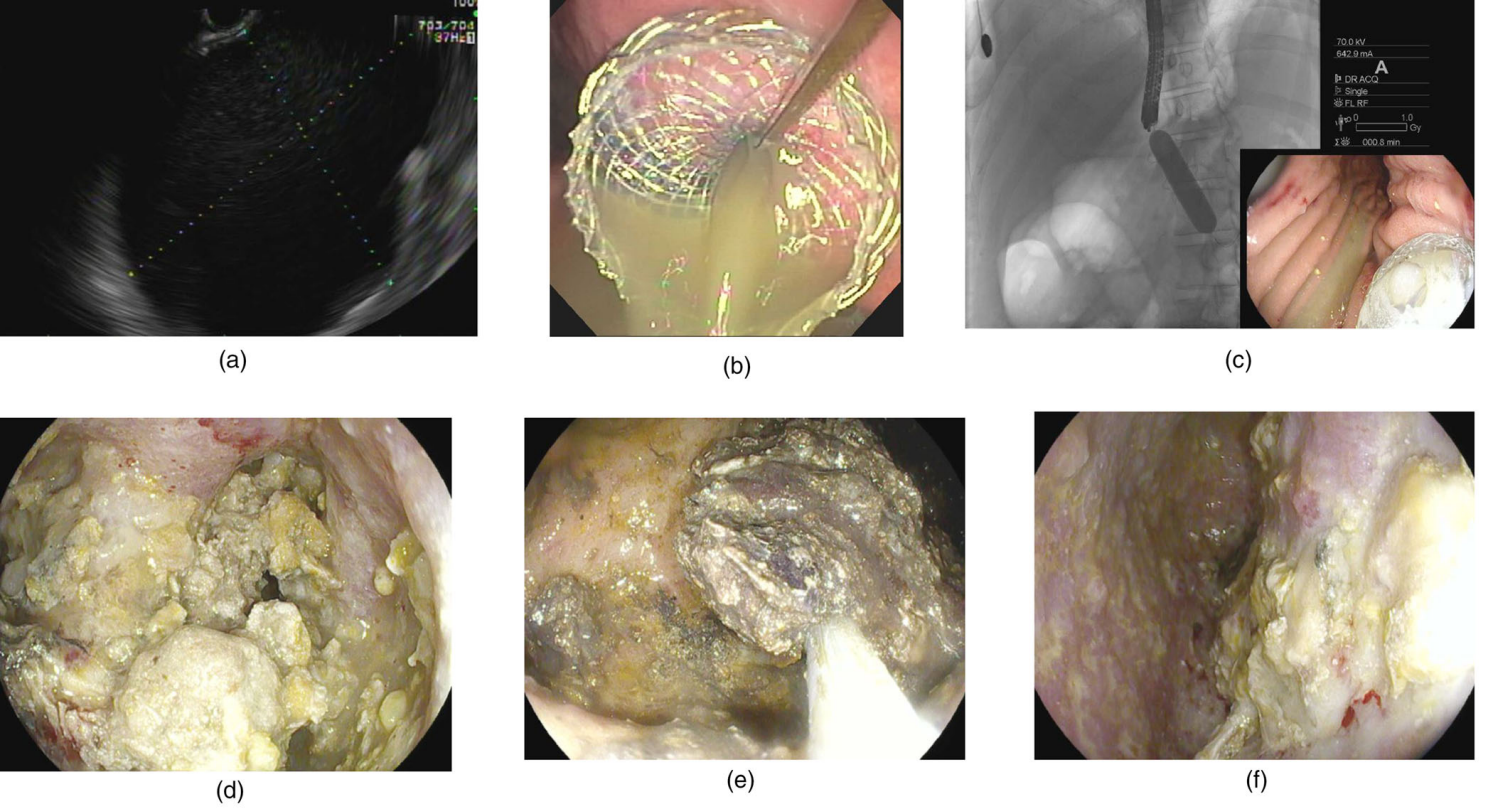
Endoscopic management of walled‐off necrosis. (a) Endoscopic ultrasound imaging of walled‐off necrosis. (b) Endoscopic visualization of lumen apposing metal stent after deployment in the cyst cavity with drainage of purulent fluid. (c) Balloon dilatation of lumen apposing metal stent after deployment under endoscopic and fluoroscopic guidance. (d) Endoscopic visualization of necrosis in the cyst cavity prior to direct endoscopic necrosectomy. (e) Direct endoscopic necrosectomy using a snare. (f) Clean cyst cavity after direct endoscopic necrosectomy
